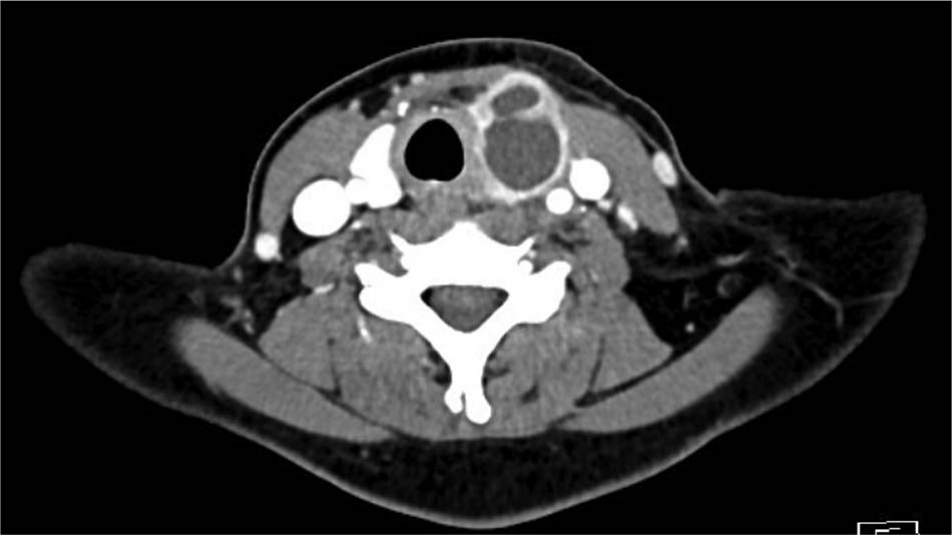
Axial portal venous phase CT of the neck demonstrating the cystic lesion in the left thyroid gland.
